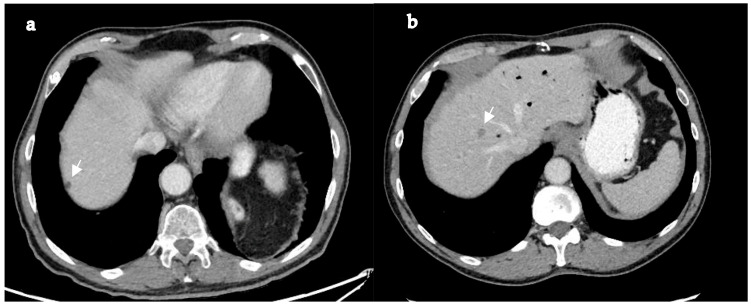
Representative axial contrast-enhanced CT images of a patient with PDAC-LOM. (a) Arrows hypoattenuating lesion in liver segment VII measuring 8 mm, consistent with a liver metastasis. (b) Arrows hypoattenuating lesion in liver segment VIII measuring 7 mm.
